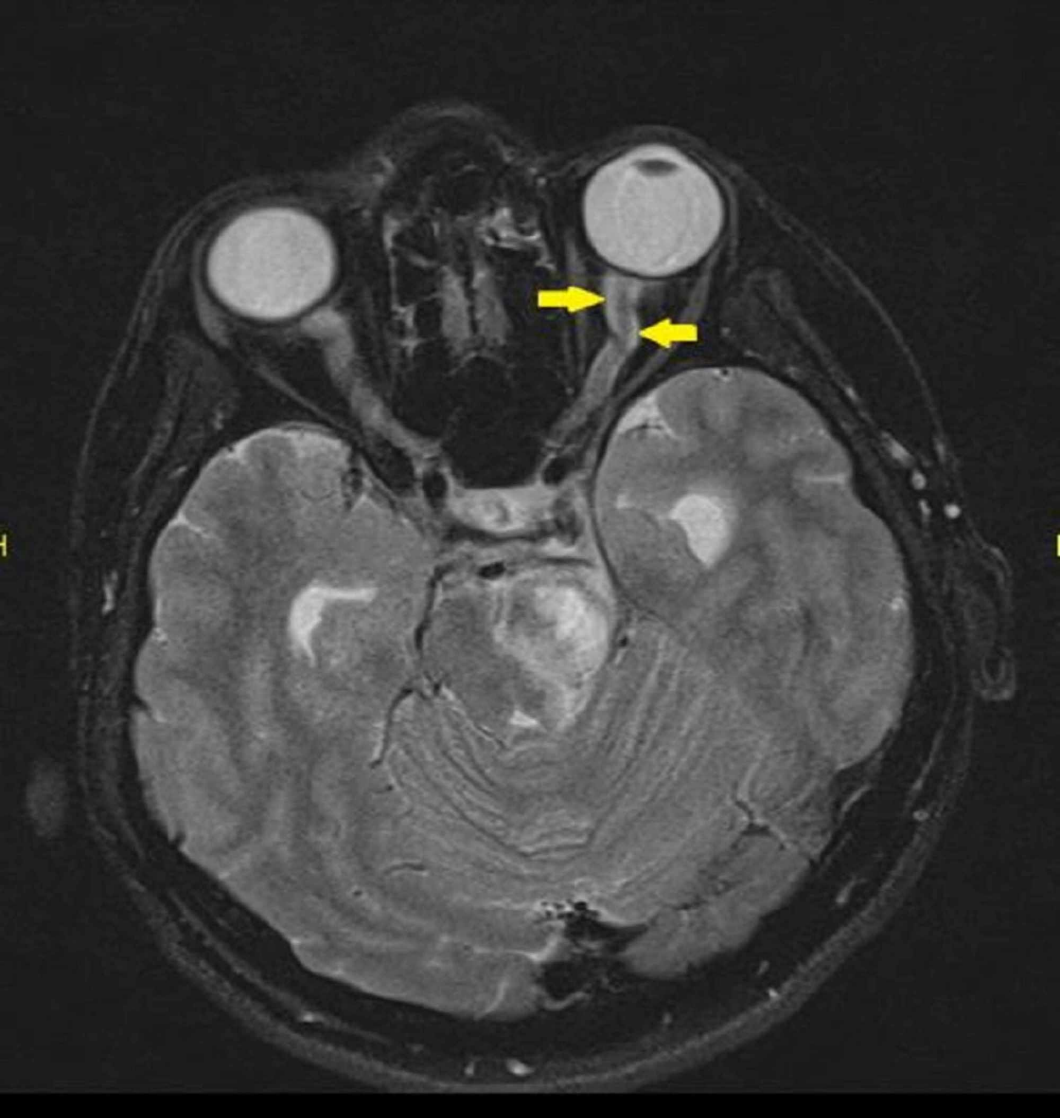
Magnetic resonance imaging (MRI) showed kinked left optic nerve with thickened optic nerve sheath
